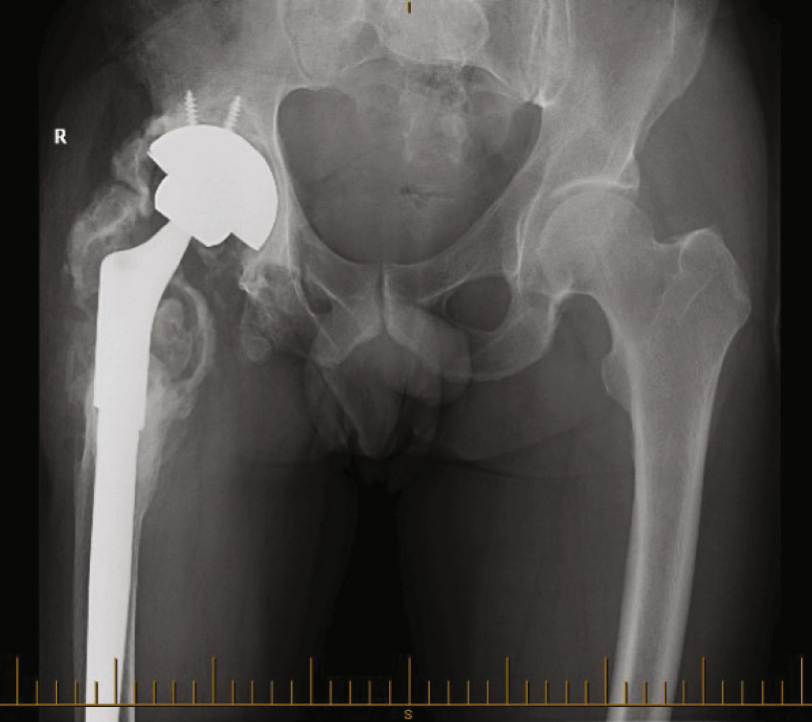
Pelvic X-ray of the patient before the 2016 operation.
